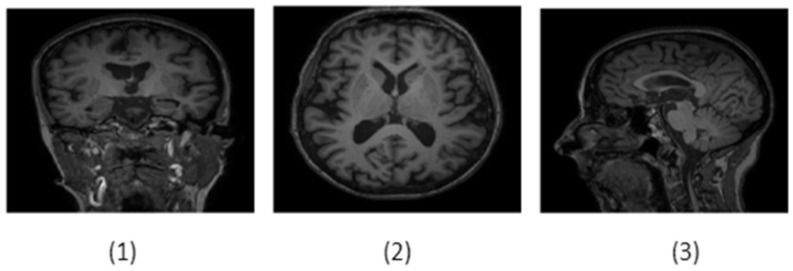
Three-plane brain MRI images from the ADNI database: (1) coronal; (2) axial; (3) sagittal.
